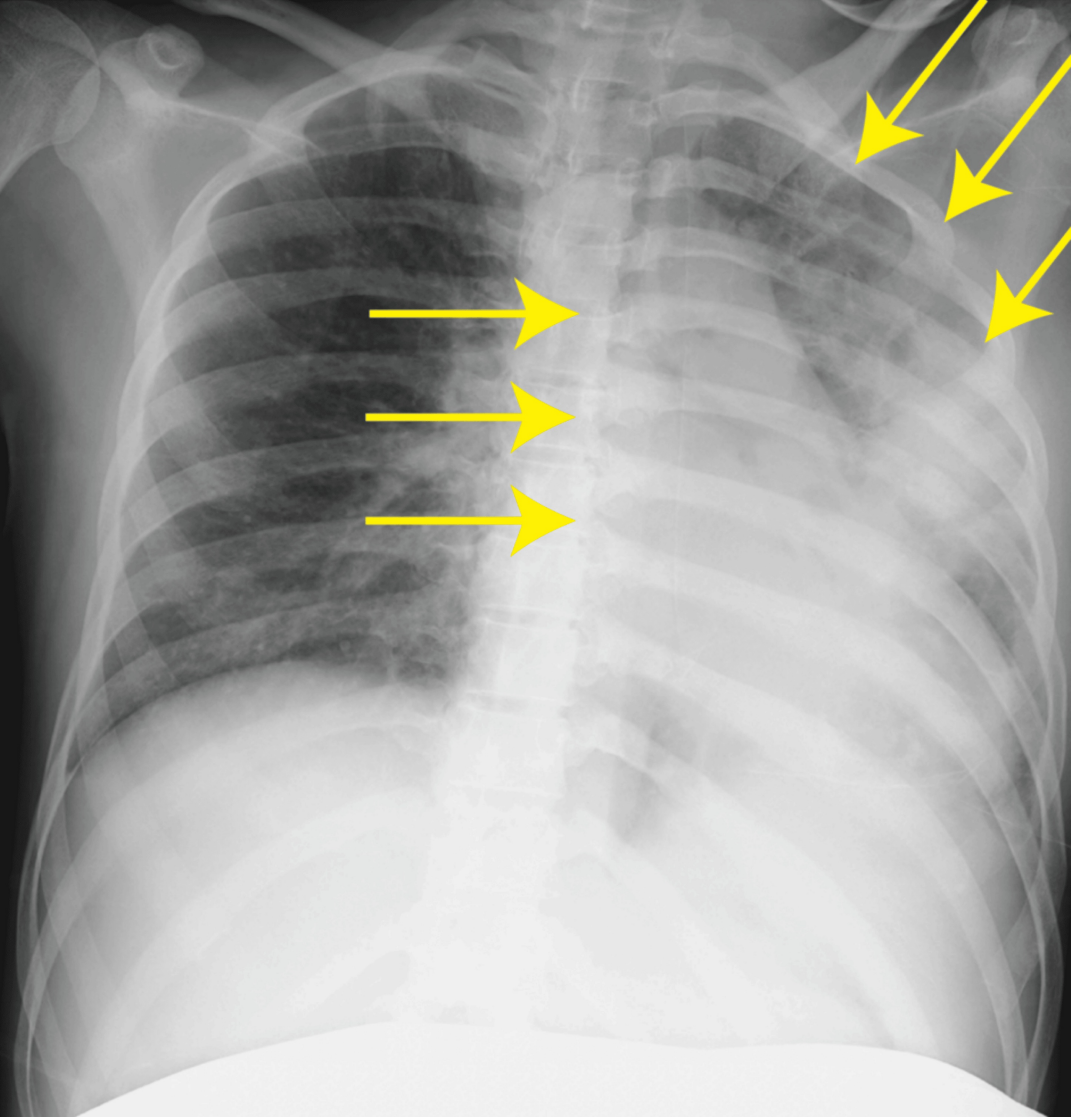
Chest X-ray imaging The area of pulmonary edema is indicated by yellow arrows.
